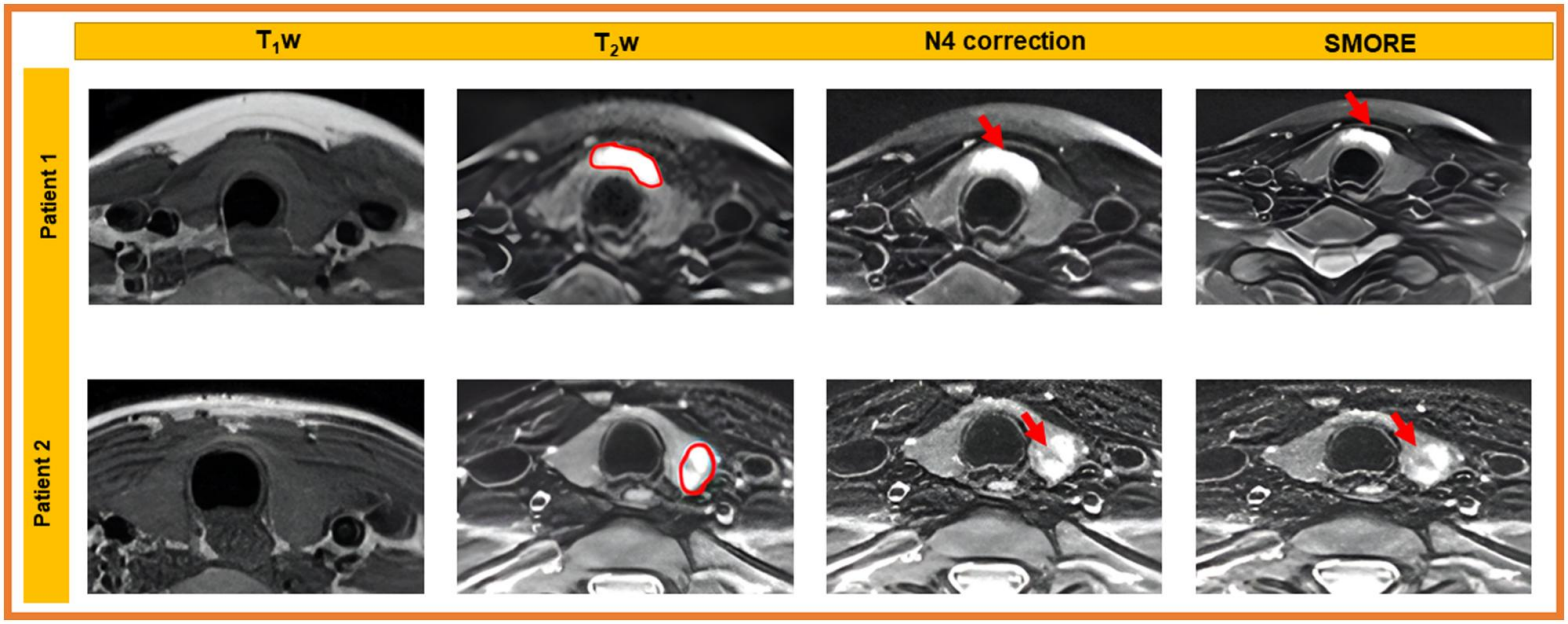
T1w images and fat-suppressed T2-weighted images for 2 patients demonstrate STIR hyperintense tumour in the thyroid isthmus (patient 1, ROI) and T2 heterogeneous tumour in the right thyroid lower pole (patient 2, ROI). Image quality for both patients is maintained between N4 bias-field corrected images and SMORE images, noting tumour visibility without significant artefacts.
